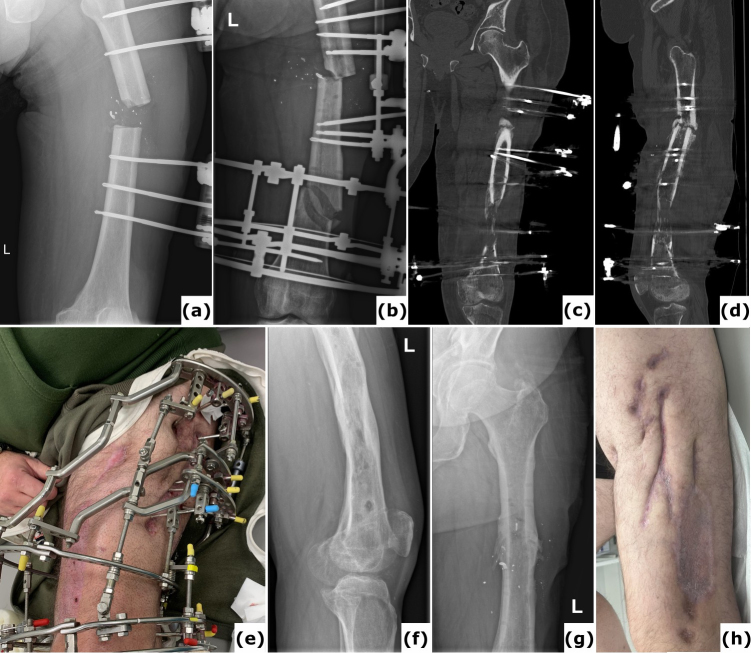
A case of polymicrobial fracture-related infection (FRI) of the femur resulting from a gunshot injury is described. A 44-year-old polytrauma patient presented with multiple injuries, including a Gustilo-Anderson type III open femur fracture of the left lower limb. (a) The patient was transferred after initial stabilization, external fixation of the left femur, and several surgical wound debridements. (b) Anteroposterior radiograph of the left femur was taken following acute shortening, distal corticotomy, and lengthening. No cultures were collected during this surgery. (c–d) Computed tomography (CT) images of the left femur at 6 months show a persistent unhealed fracture site along with a distal distraction site that is not fully consolidated. Due to the presence of suggestive signs of FRI (local redness, swelling, and elevated serum inflammatory markers), local debridement of the fracture site was undertaken. Cultures grew *Klebsiella pneumoniae* and *Enterococcus faecalis*, for which the patient received systemic antibiotic therapy (6 weeks). (e) Clinical image of a classic ring fixator. (f–g) Lateral and anteroposterior radiographs after removal of the external fixator at 2 years demonstrate consolidation of the fracture and the distal distraction site. Because of continued pin site infections, local redness, and elevated serum inflammatory markers, the intramedullary canal was reamed and cultures were obtained, all of which were positive for *Staphylococcus aureus*. Again, the patient received systemic antibiotic therapy (6 weeks). (h) Clinical images of the lower limb 1 year after removal of the external fixator show significant scarring of the left thigh from the pin tracts. Knee flexion was limited to 10°. This case shows that long-term external fixation is not harmless and can be associated with several potential complications, such as joint contracture, scarring from the pin tracts, and severe chronic infections.
